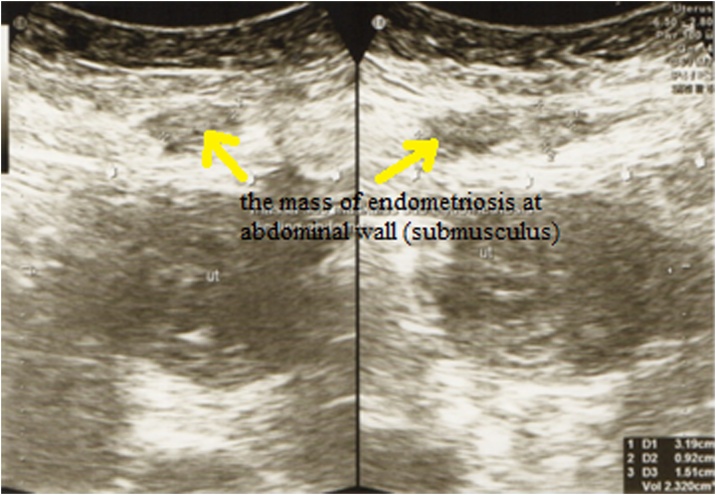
Ultrasound examination results.
